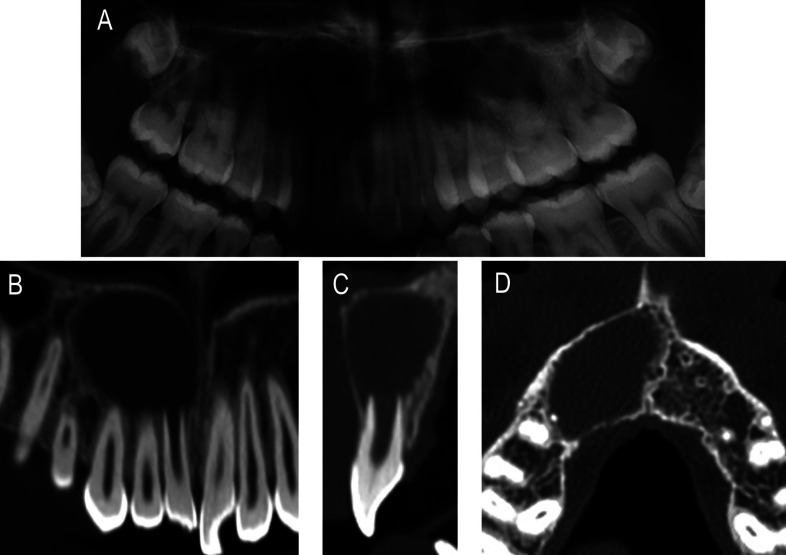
A) Pre-op orthopantomography ilustrating a extensive radiolucent lesion in the upper right maxilla related to the apices of 1.1, 1.2, and 1.3. (B-D) Selected images of computed tomography B) Coronal slice showing the lesion affecting from 1.1 to 1.5 C) Sagital slice show the lesion in relation with open apex 1.1 D) Axial slice show extensive lesion occupying bone marrow in the upper right maxilla and bulging cortical bone plates.
